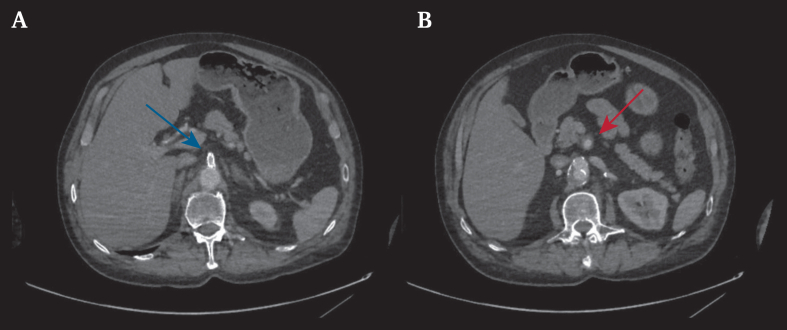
Computed tomography angiography 11 months after surgery. The blue arrow highlights the superior mesenteric artery stent. The red arrow highlights the superior mesenteric artery.
